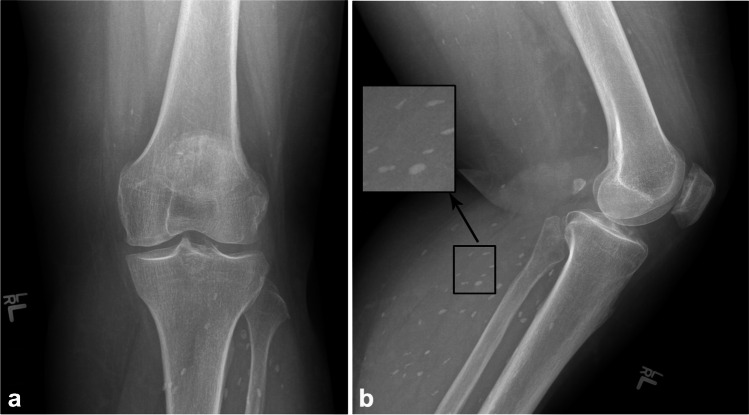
Sixty-year-old woman with left knee pain. AP (a) and lateral (b) radiographs demonstrate numerous intramuscular “rice grain” calcifications, compatible with cysticercosis from prior ingestion of *Taenia solium* eggs via fecal–oral transmission
